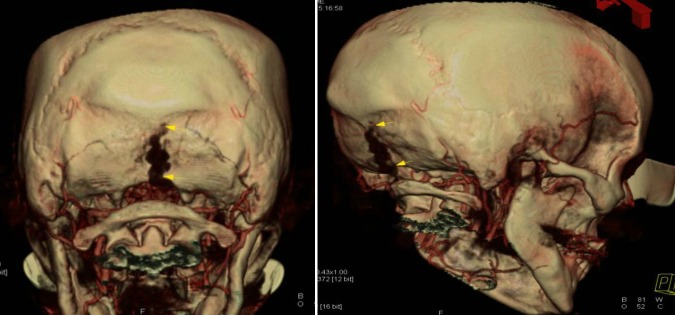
3D reconstruction of the CTA showing the occipital emissary vein course to the suboccipital venous plexus.
